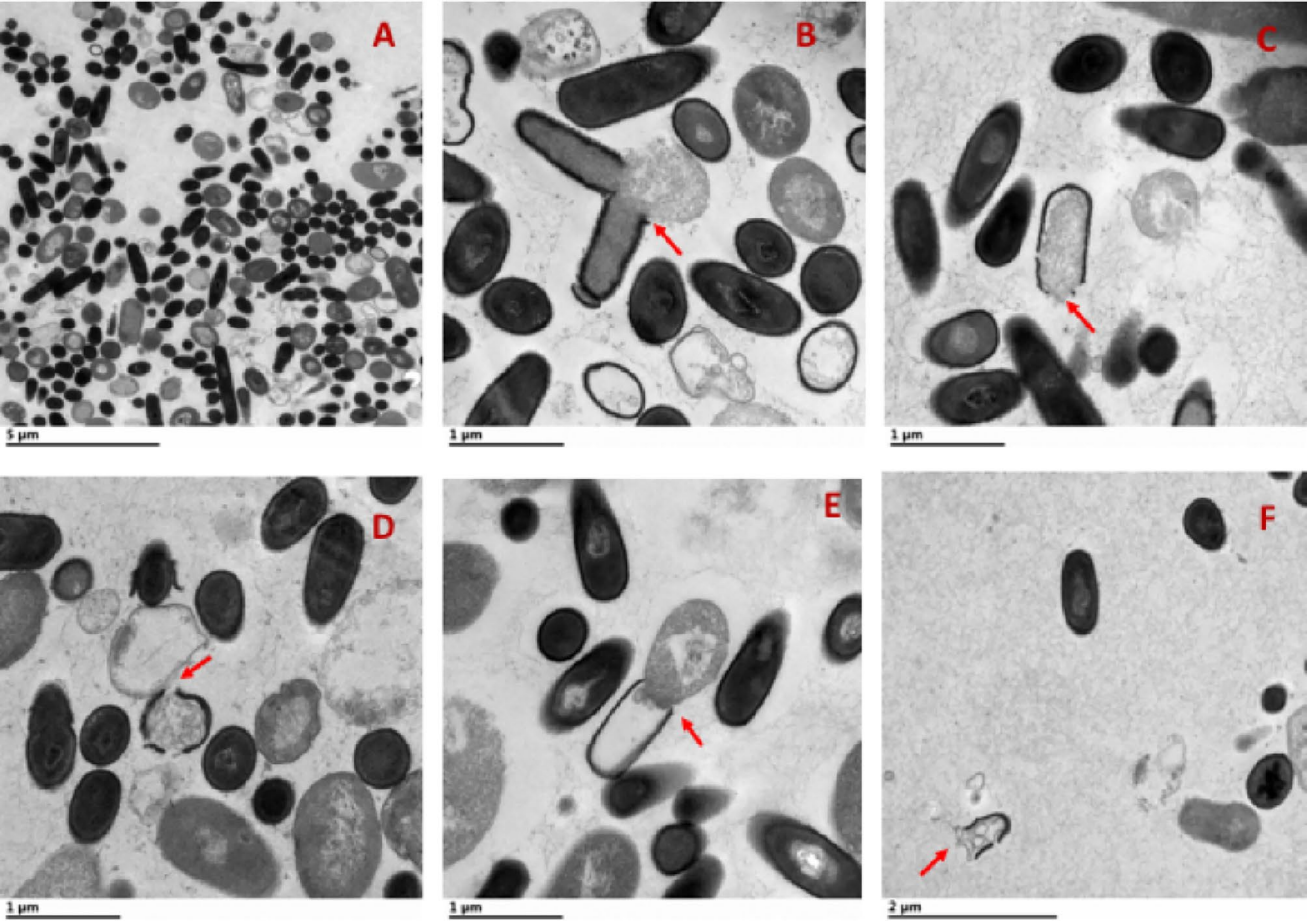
TEM show single or multiple pore formations (red arrow) on *E. coli* due to ultrasound and cecropin P1 treatment. (A) control, (B) 14 kHz, (C) 22 kHz, (D) 47 kHz, E, and F: a combination of ultrasound (22 kHz) and cecropin P1.
